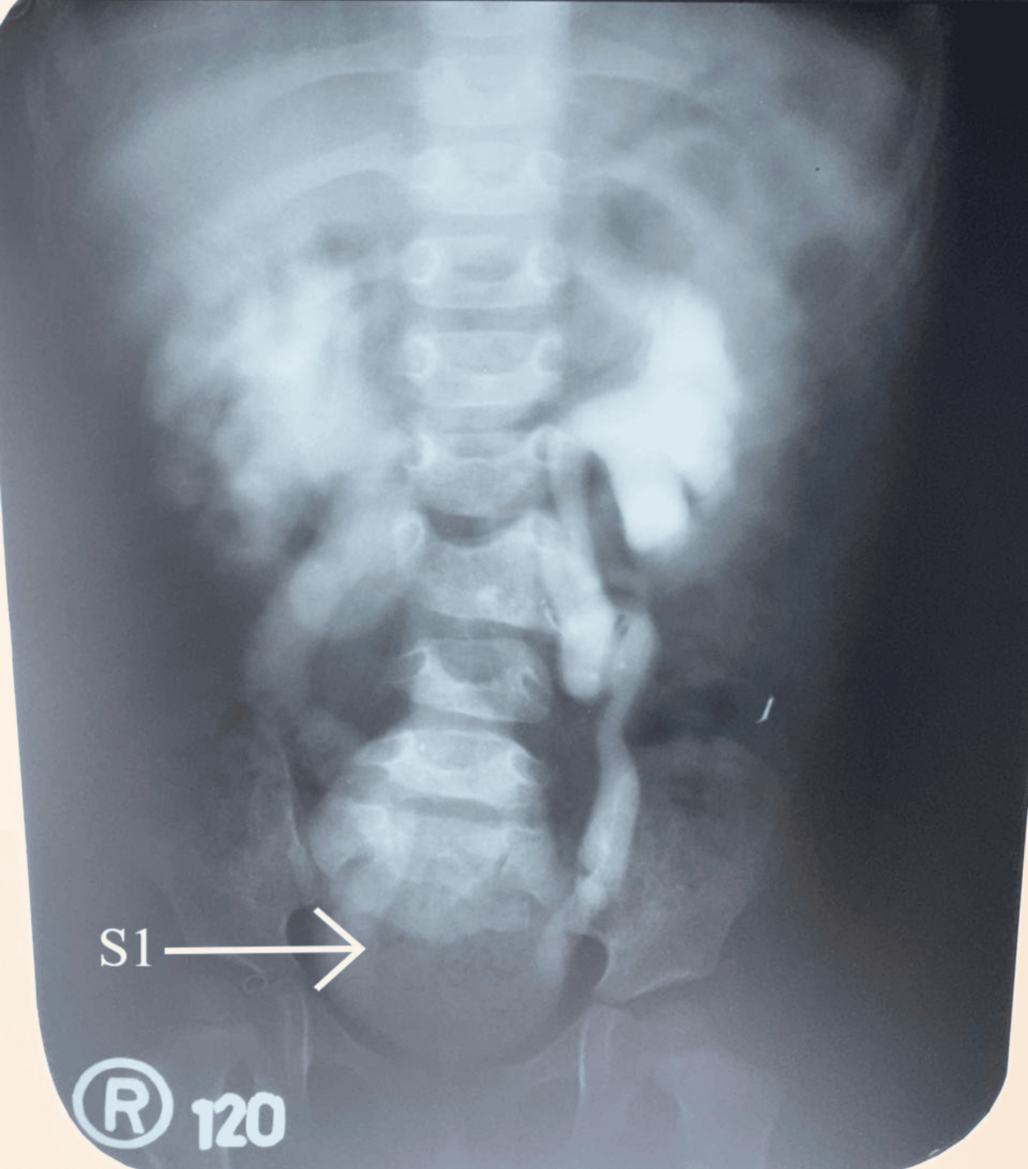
Pelvic X-ray (PA view) showing distal sacral agenesis Pelvic X-ray (anteroposterior view, bladder filling phase) obtained in early childhood demonstrating non-visualization of the distal sacral and coccygeal vertebrae. The arrow indicates the abrupt truncation of the sacrum, while the S1 vertebral segment is clearly delineated for reference. Film coverage was adequate, and there was no obscuration by bowel gas. These findings are consistent with distal sacral agenesis. PA: posteroanterior
